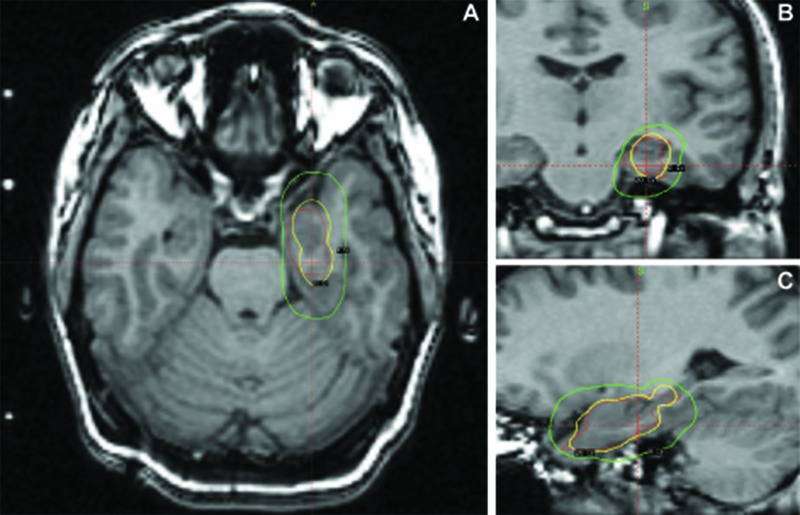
Preprocedural MRI for radiosurgical planning with superimposed dosimetry data. Axial (A), coronal (B) and sagittal (C) non-contrast T1-weighted images obtained for radiation planning. Eighty percent of the target volume (red) was covered by the 20 Gy isodose surface (yellow). The peripheral target volume covered by the 8 Gy isodose surface was also shown (green).
